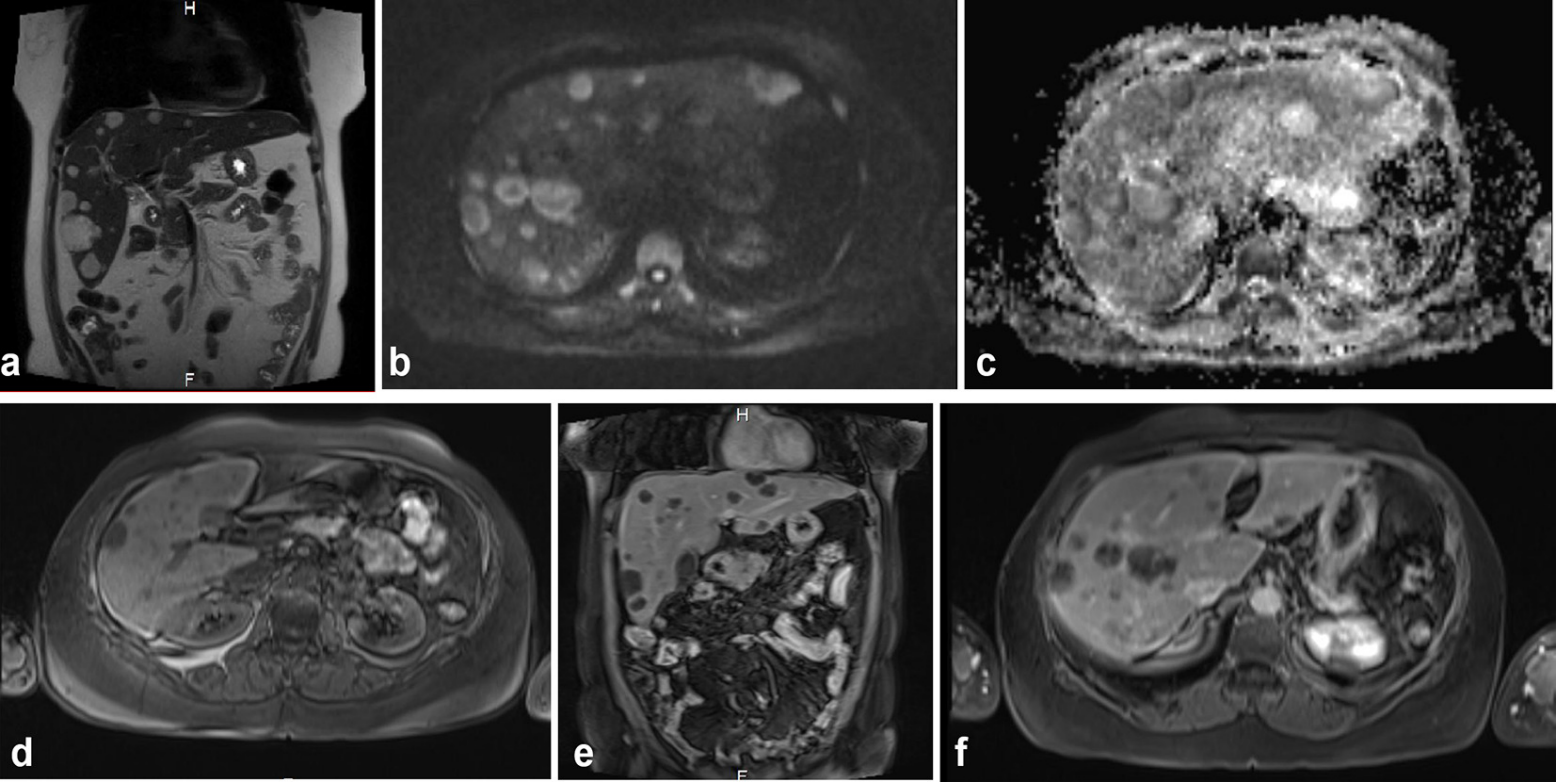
MRI showing a similar pattern of enhancement. (a) Coronal sequence, *T*_2_ Half Fourier Single Shot Turbo-spin Echo, (HASTE): normal-sized liver with multiple, hyperintense lesions in all liver segments; (b and c) diffusion sequences: lesions show restriction predominantly in the periphery; (d) *T*_1_ fat suppression without contrast media: hypointense lesions in the liver; (e) *T*_1_ coronal sequence in portal phase: the lesions remain without significant uptake of contrast medium; (f) Axial *T*_1_ late phase, 5 min after administration of contrast medium: the lesions with some small zones of central uptake.
